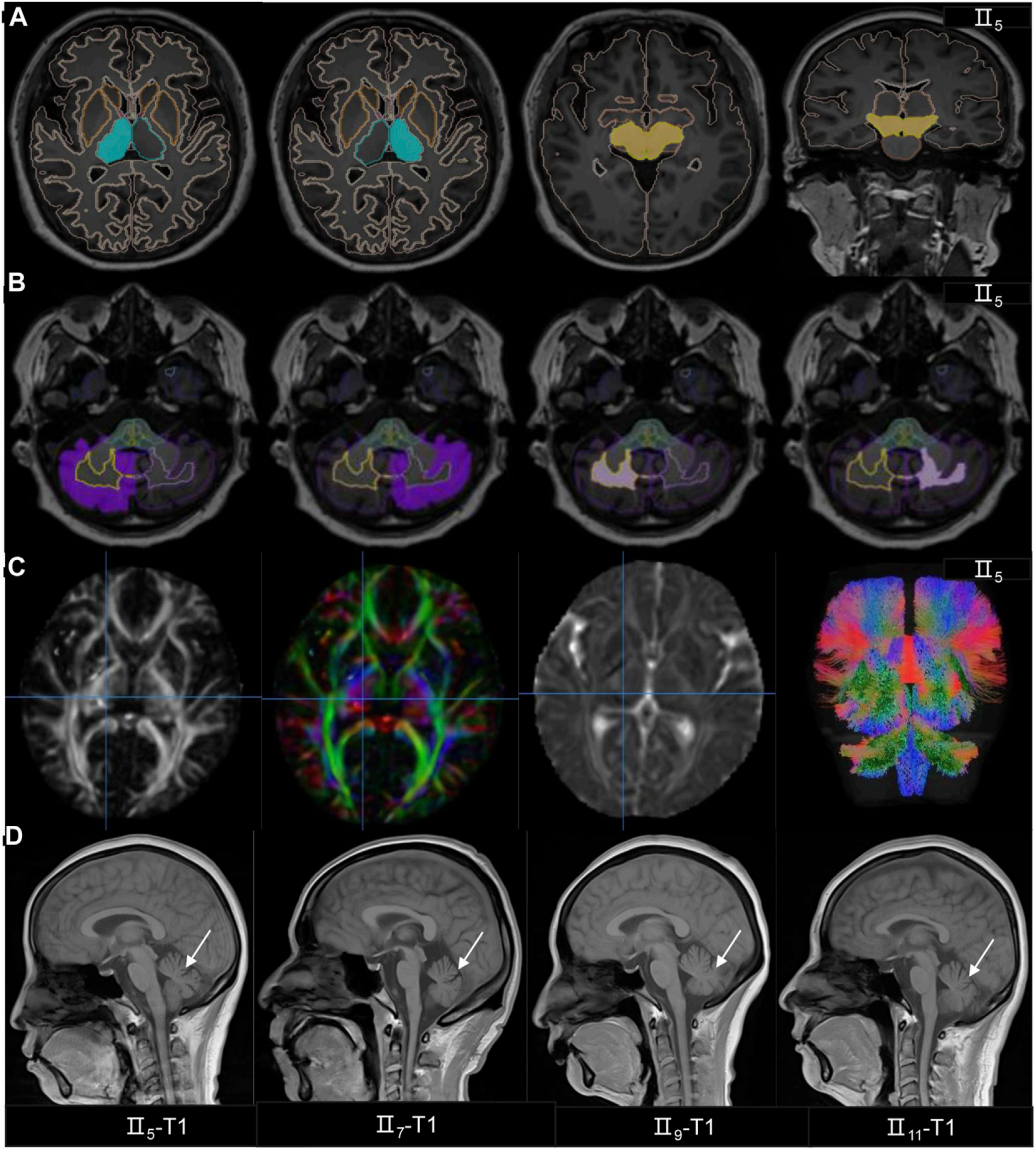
The MRI findings of the SCA family. (A–B) The 3D-T1 analysis showed the patient’s (II_5_) midbrain and thalamus volumes were normal, but the volumes of gray matter and white matter volumes in the cerebellum had decreased. (C) The patient’s (II_5_) DTI analysis showed the FA, DEC, AD and white matter bundles followed by fiber quantification. (D) MRI T1 images are in order II_5_,II_7_,II_9_ and II_11_, and T1 indicates atrophy of the cerebellum.
